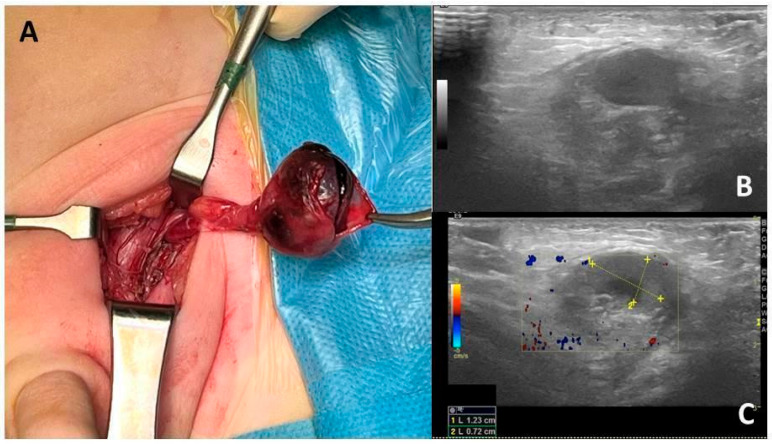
(A) Intraoperative appearance of necrotic left testicle. The albuginea is opened revealing necrotic parenchyma. (B,C) Ultrasound appearance of the testicle: the iso/anechoic image reveals no vascular signal and an anechoic colliquative necrosis area.
